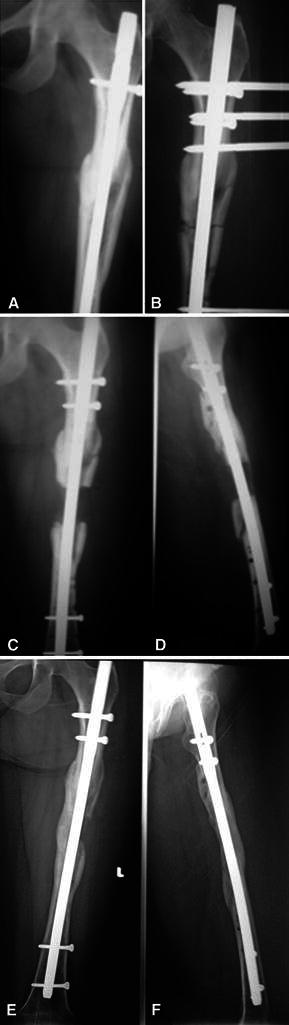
Serial X-ray of a case of lengthening over nail: a before lengthening. b After operation. c, d After removal of fixator. e, f After full consolidation
